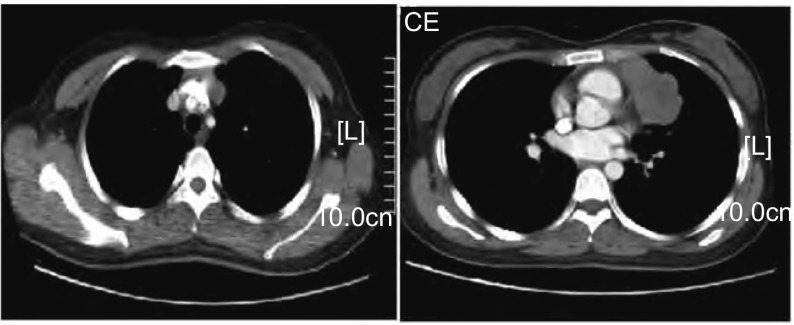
胸部强化CT示：前纵隔不规则肿块，侵犯左头臂静脉，并突向左上叶，增强检查后肿块呈明显不均匀强化，其内可见片状无强化坏死区。 The chest enhancement CT scan revealed an irregular anterior-mediastinal mass with invasion of the left brachiocephalic vein and the left upper lobule of the lung. The bulky lesion was obviously unequal strengthening with contrast-enhanced CT scans, and it was visible flake necrotic area.
